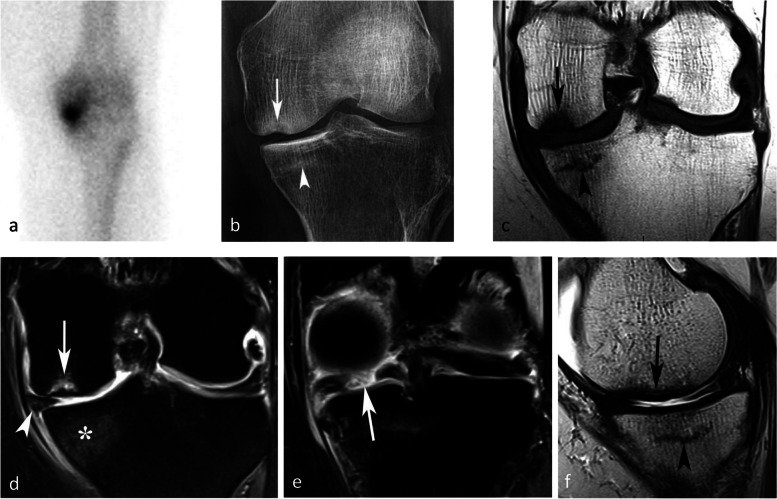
Subchondral insufficiency fracture (SIF) and subchondral insufficiency fracture complicated with osteonecrosis (SIF-ON). a Bone scan in a woman who presented with sudden onset of pain for several weeks, showing intense uptake in the medial femorotibial compartment. b Two months later, radiograph shows focal collapse of the inferior pole of the medial condyle (arrow) with sclerosis of the adjacent cancellous bone, and a sclerotic horizontal band parallel to the medial tibial plateau (arrowhead). c MR shows a focal very low signal intensity on coronal T1-weighted image in the medial condyle lower pole (arrow) and a thin low signal intensity horizontal band under the medial tibial plateau (arrowhead). Fat-suppressed T2-weighted image (d), showing heterogeneous signal in the condylar lesion (arrow) and a bone marrow moderate high signal under the tibial plateau (asterisk). e A T2-weighted MR posterior coronal image shows a wide radial rupture of the medial meniscus (arrow), causing extrusion of the meniscus middle portion (arrowhead in d). f Sagittal T2-weighted image shows low signal intensity in a thick and extended subchondral bone marrow area adjacent to the condylar surface (arrow), corresponding to an area of degraded bone marrow. Under the tibial plateau, the thin horizontal band with a low signal intensity (arrowhead) is surrounded by an almost normal marrow signal intensity. These aspects are typical of a SIF for the tibial plateau and a SIF-ON for the condyle
